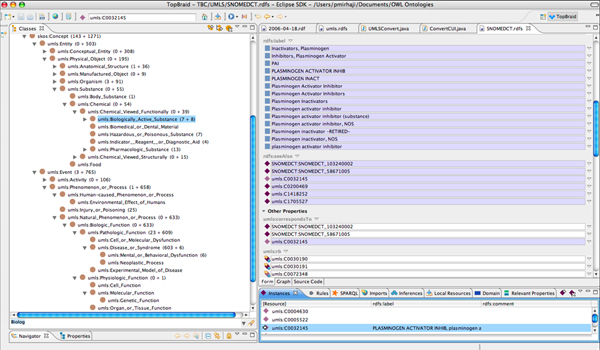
Snapshot of the SKOS representation of a biomedical concept. The right side panel represents the SKOS:Concept related to "Plasminogen Activator Inhibitor", synonymous terms associated with it, two distinct SNOMED-CT concepts and a CUI that correspond to it, several other similar or associated concepts (rdfs:seeAlso) from UMLS, and its multiple broader concepts. The Semantic Type (umls:Biologically_Active_Substance) and other relevant classification based on the UMLS Semantic Network are presented in the left panel.
